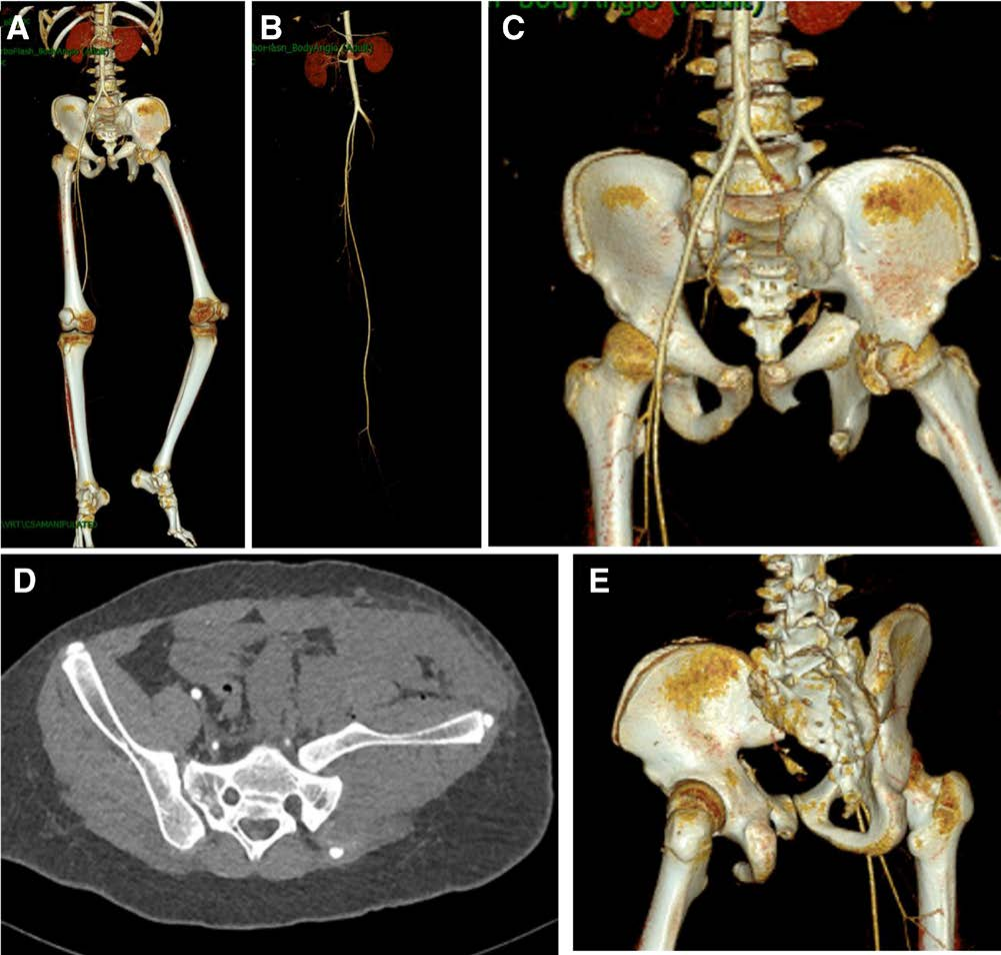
(A) Overall view of CTA 3D reconstructed image showing pelvic fracture (type C1.2), left complete anterior dislocation of the sacroiliac joint, and left acetabular fracture. (B) Angiographic shadow of CTA 3D reconstructed image showing left internal and external iliac arteriovenous dissection. (C) Anterior view of CTA 3D reconstructed image. (D) Cross section of CT image. (E) Posterior view of CTA 3D reconstructed image.
